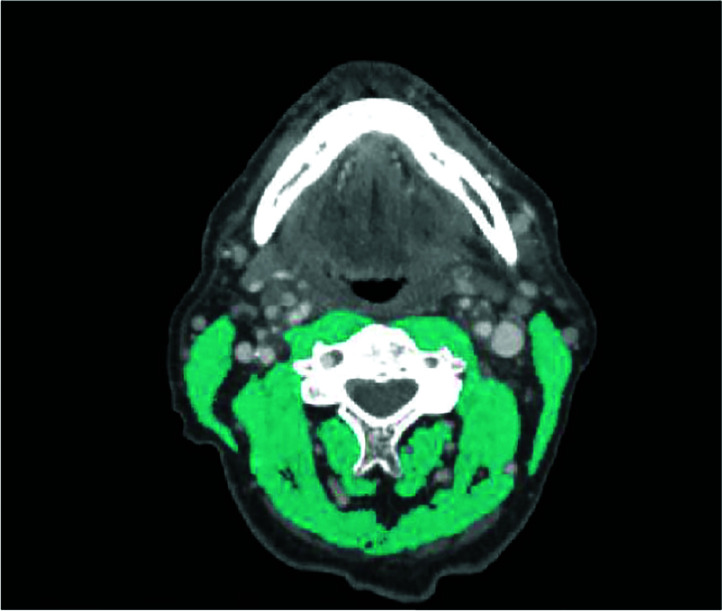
Delineation of skeletal muscle tissue on transversal CT imaging at the level of C3. A Hounsfield Unit window of -29 to +150 was used to accentuate skeletal muscle tissue.
